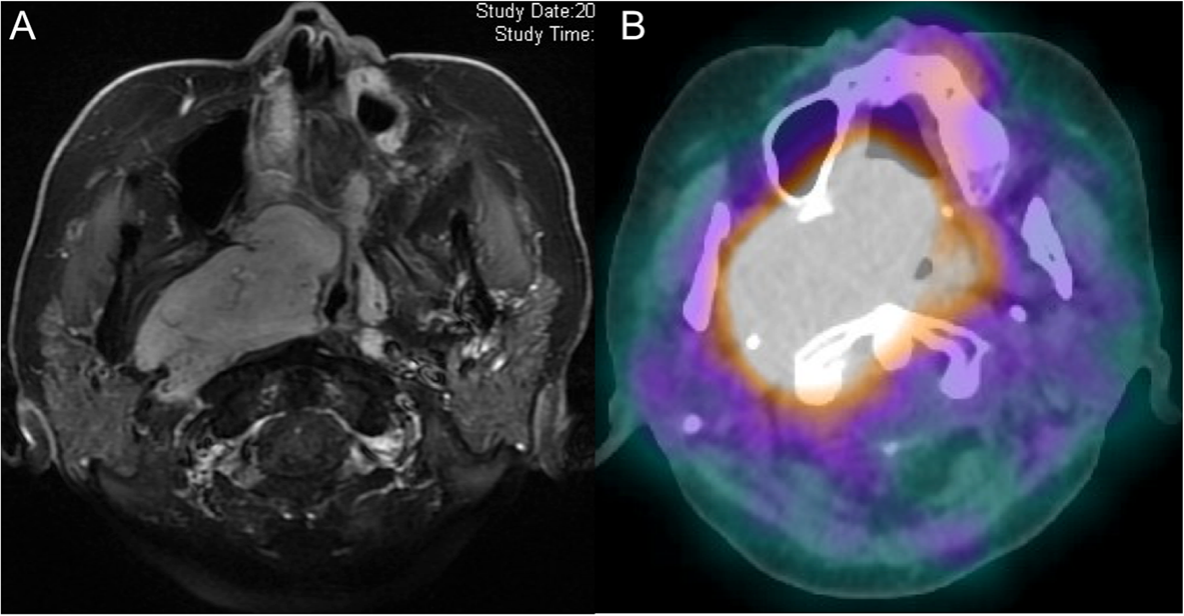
a T1-weighted contrast-enhanced magnetic resonance imaging of an axial image showed an enhancing mass in the right nasal cavity and ethmoid sinus. b Somatostatin receptor scintigraphy (Octreoscan) showed strong tracer uptake consistent with the tumor
